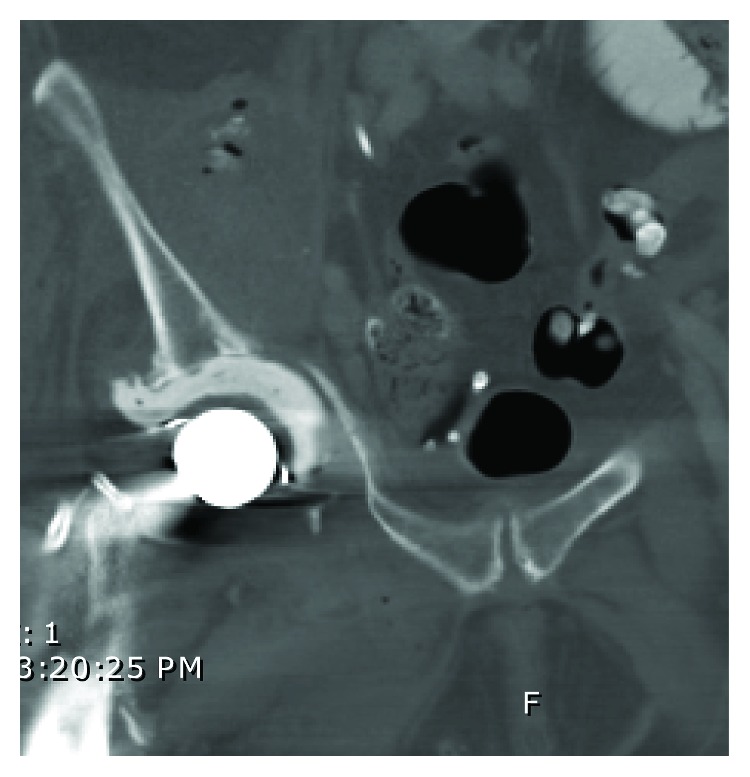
Stage 1: postoperative CT scan.
